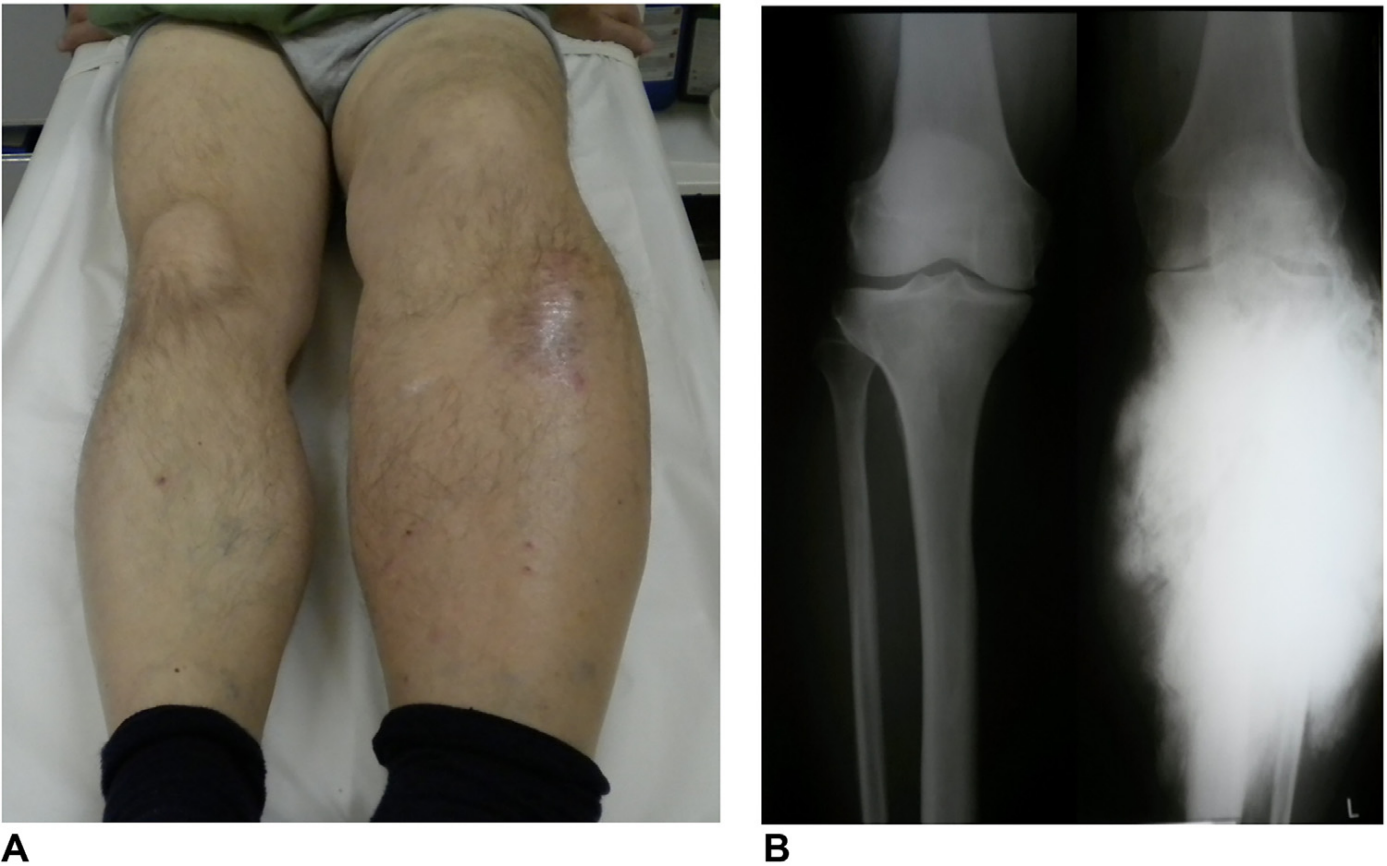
Photograph of the lower limb at the time of presentation to our institute (A). Leg circumference of the left proximal part was plus 10 cm compared to the right leg. Plain radiograph shows clear progression of the calf calcification (B).
